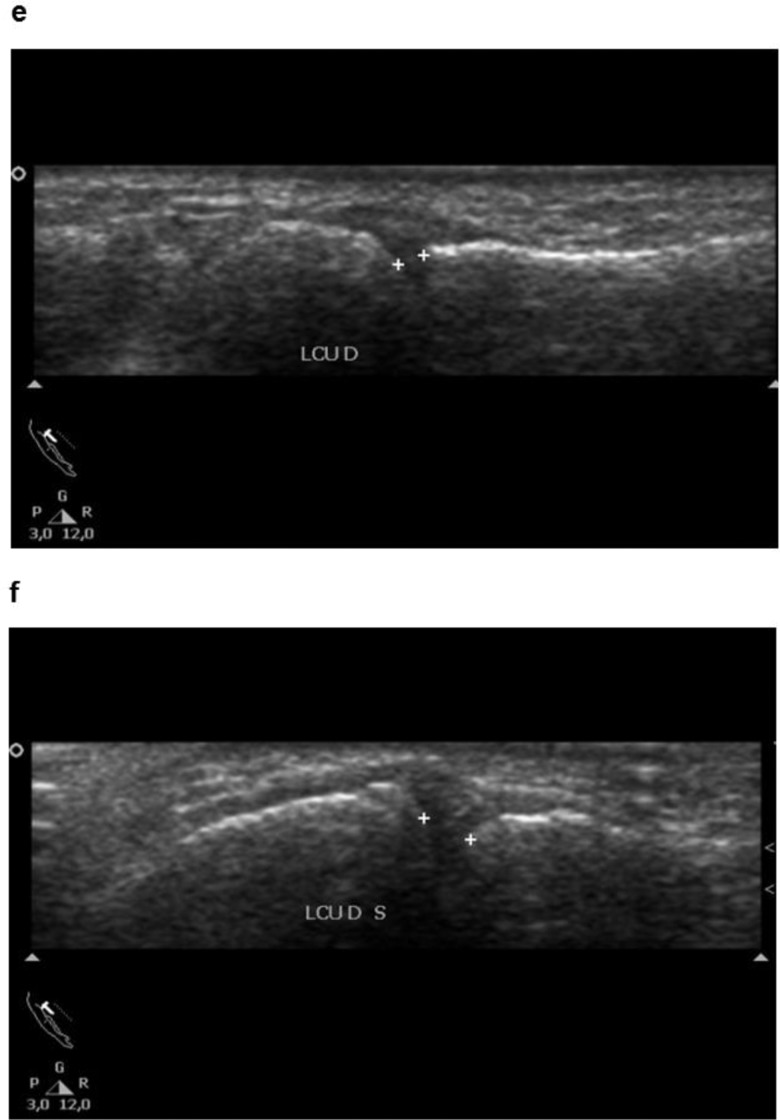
Ultrasonographic images showing the articulation opening of the metacarpophalangeal joint in the resting position (e) and under stress (f).
